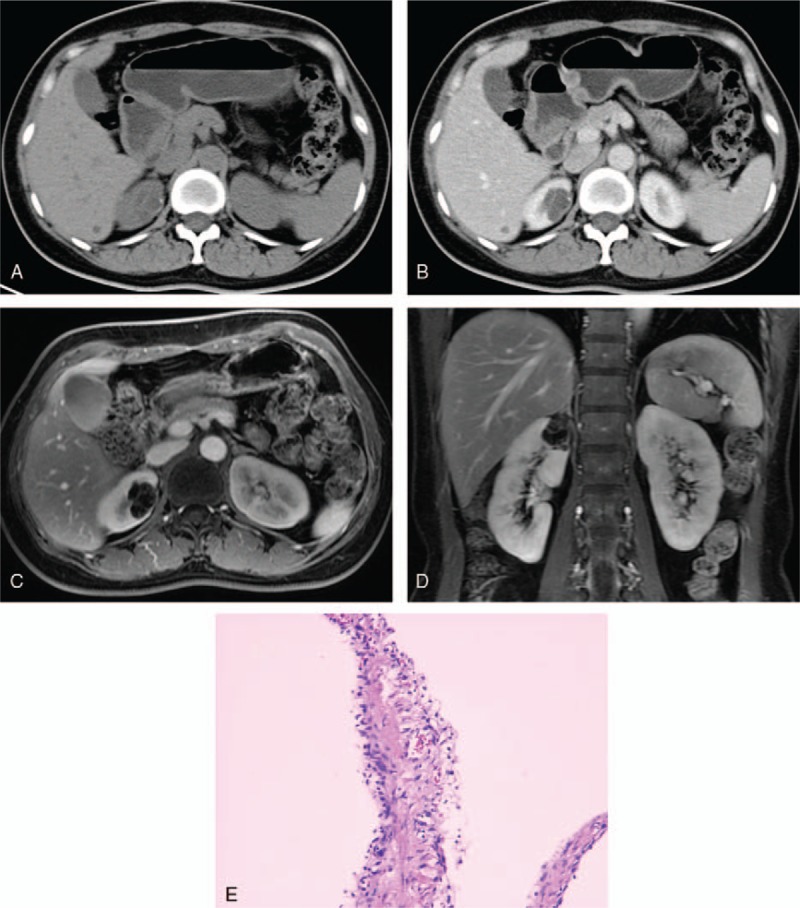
A 38-year-old woman with a cystic mass at the upper pole of the right kidney. (A, B) Axial plain and contrast-enhanced CT scans demonstrated a complex cystic mass with a few minimally thickened septa with perceived enhancement, and a short segment of slightly thickened calcification was depicted on the wall, was consistent with a category IIF cyst. (C, D) Axial and coronal gadolinium enhanced fat-suppressed T1-weighted MR image showed many septa, some of the septa were minimally thickened and others were confluent and had the gross thickening typical of category III lesions. (E) At surgery, a benign cyst was found. CT = computed tomography.
